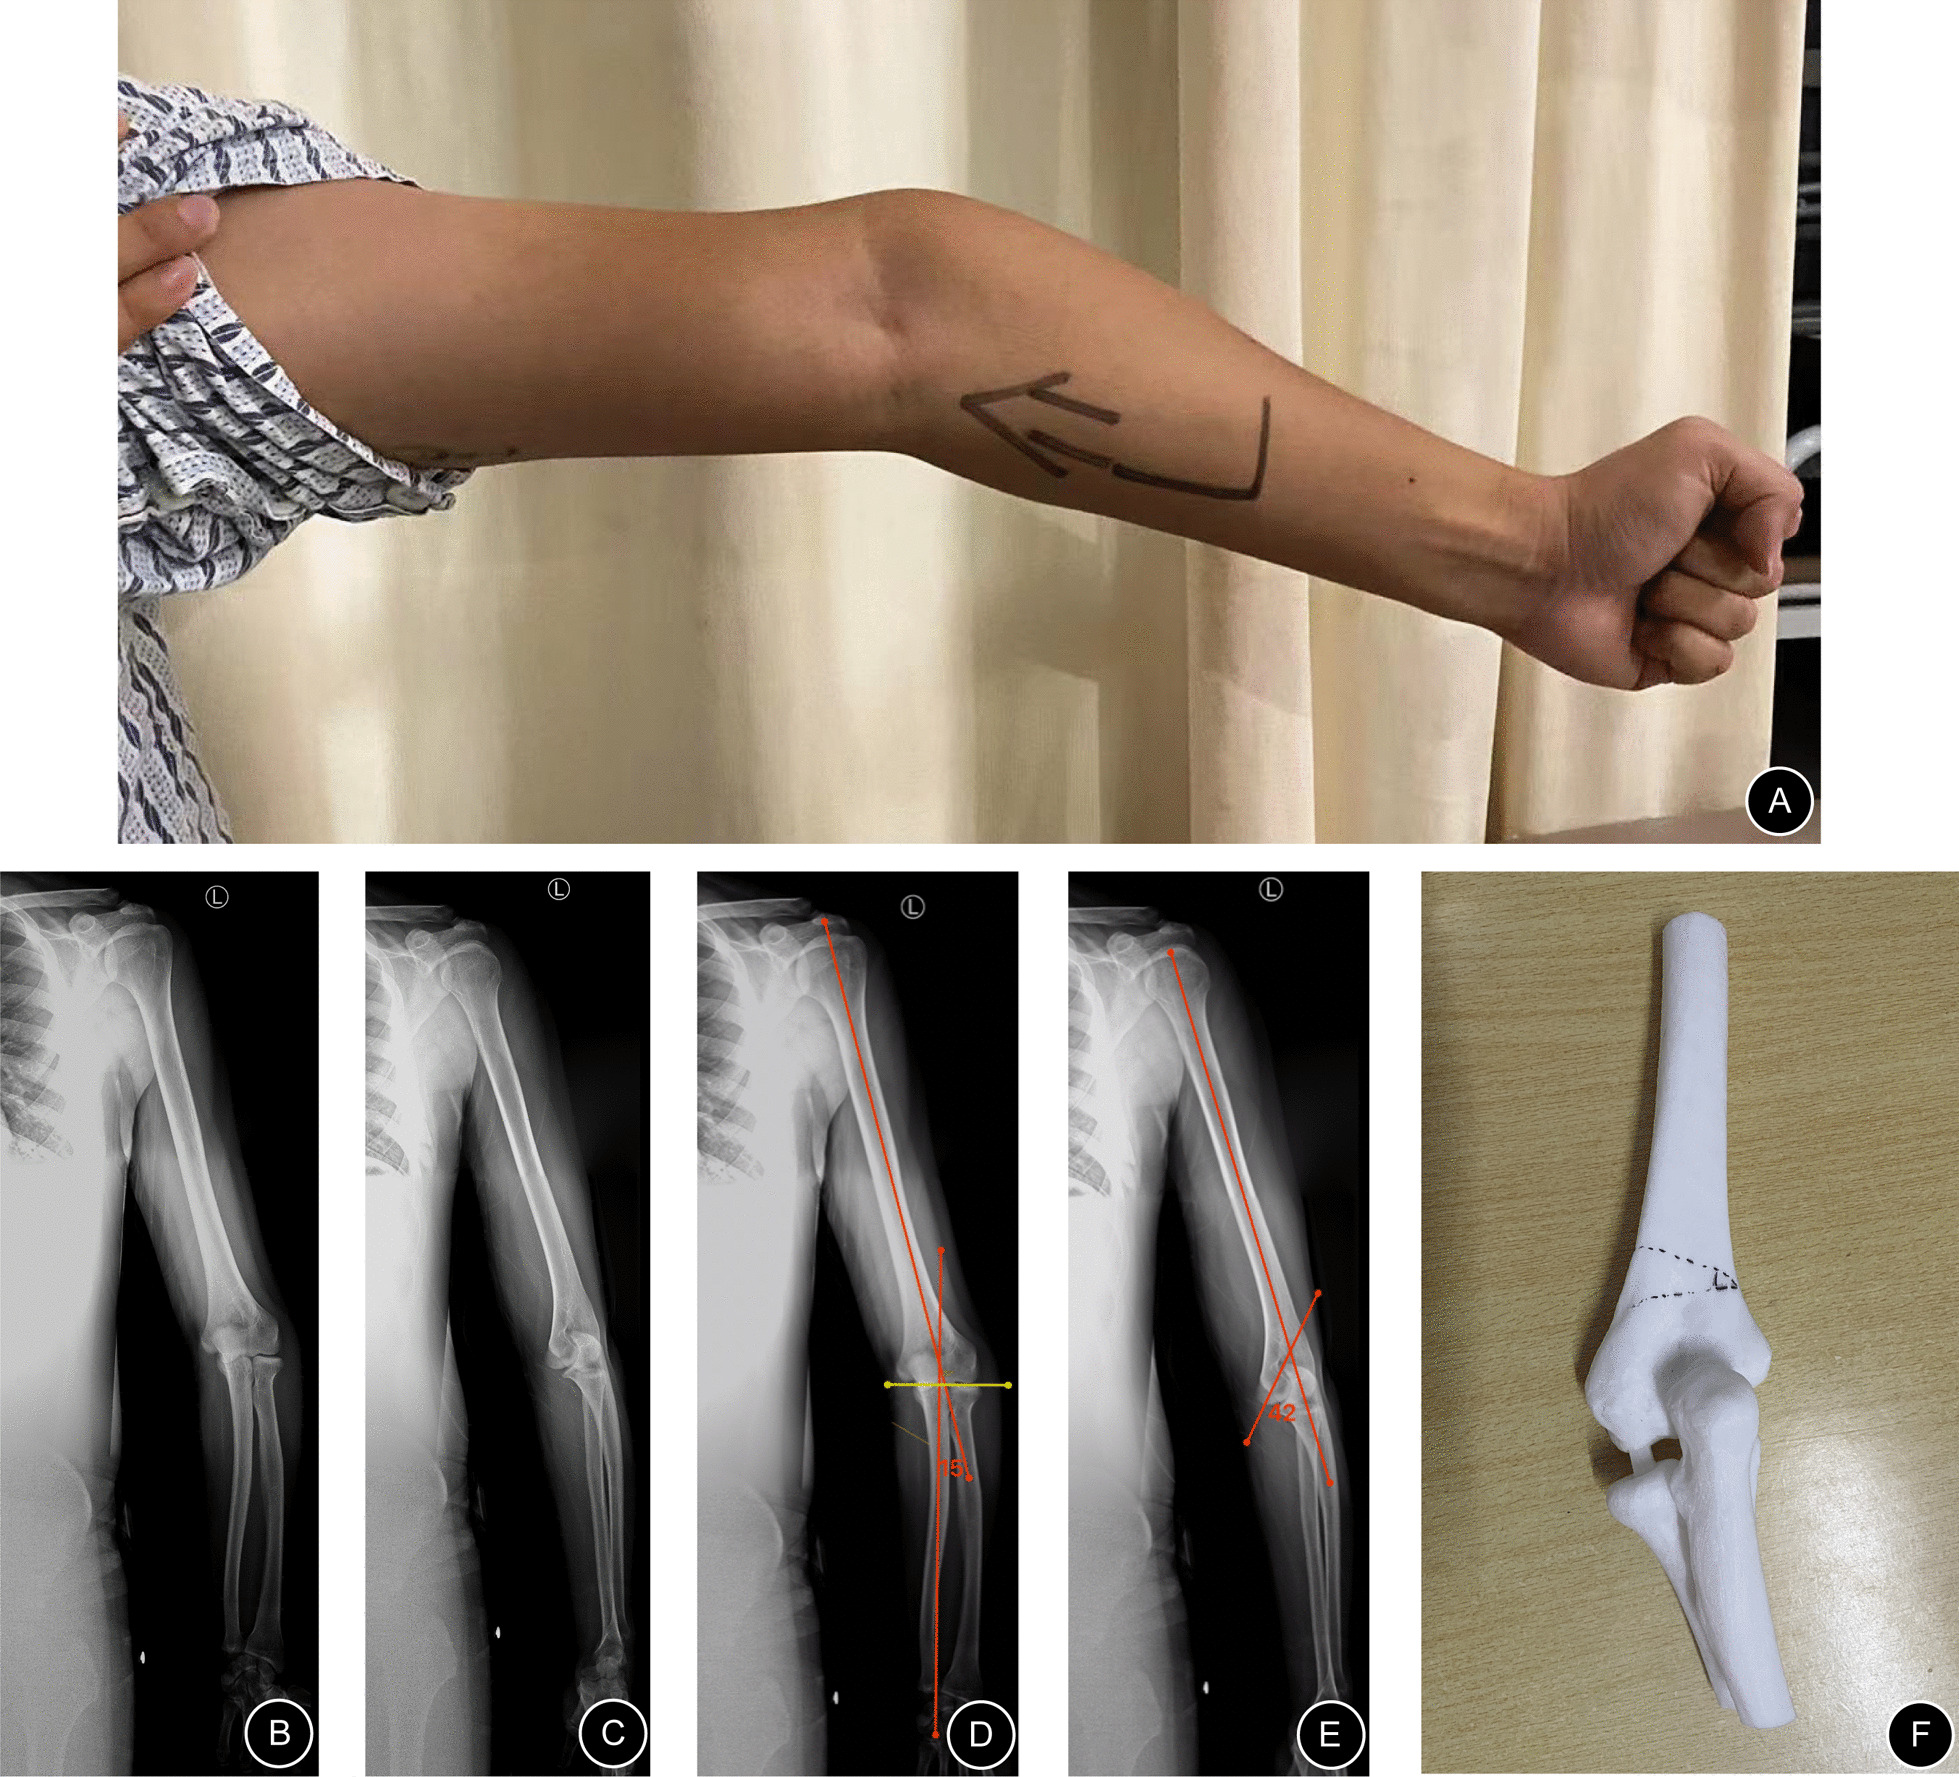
A 24-year-old man presented with a left cubitus varus deformity (Case 5). His chief complaint was mild pain with limited motion of the elbow: A Preoperative appearance. B, C Preoperative anteroposterior and lateral radiographs. D, E Preoperative deformity analyses show varus of approximately 15° and anteversion angle of the distal humerus of approximately 42°. F Osteotomy plan with the help of a 3D model
